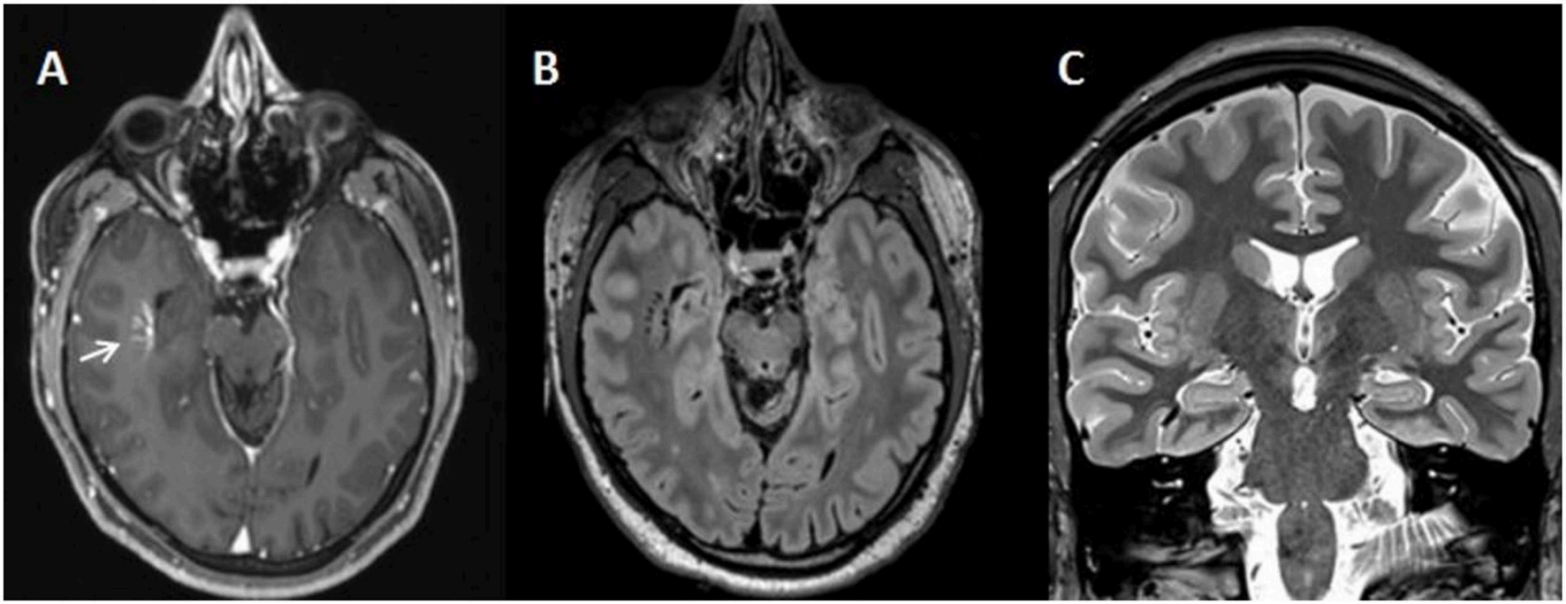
Cerebral magnetic resonance imaging (cMRI) showing an isolated uncomplicated developmental venous anomaly in the right temporal lobe (white arrow) on the contrast enhanced initial axial MPRAGE (A) and on the 2 year follow-up epilepsy specific high-resolution T2-weighted axial reformatted 3D-FLAIR (B) and coronal T2-weighted STIR (C) images. cMRI scans were acquired on a Siemens 3T scanner TRIO (A) and PRISMA (B,C).
